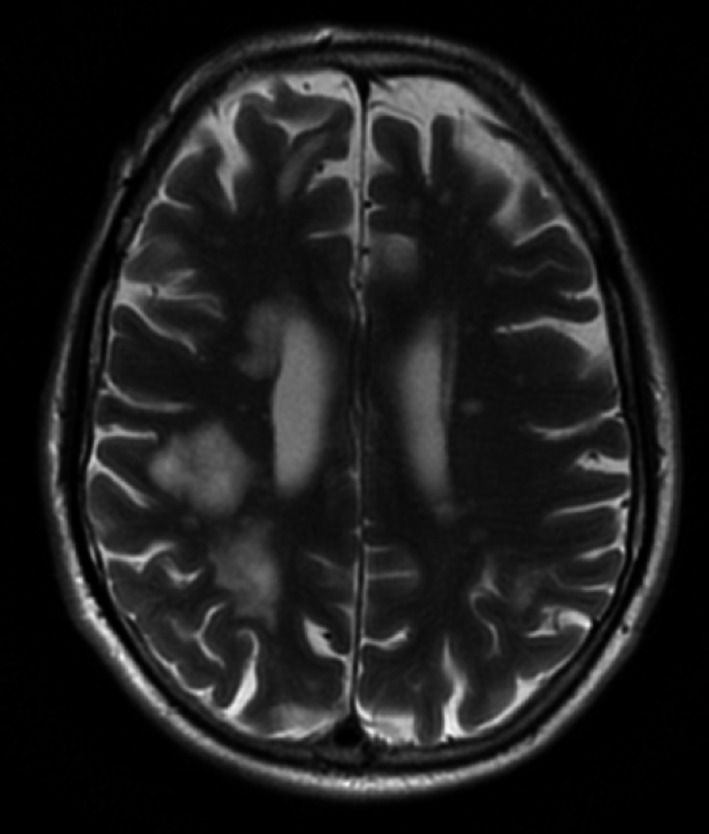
Magnetic resonance imaging (MRI) repeated one month after the MRI shown in Figure 1 shows interval progression in lesion size
